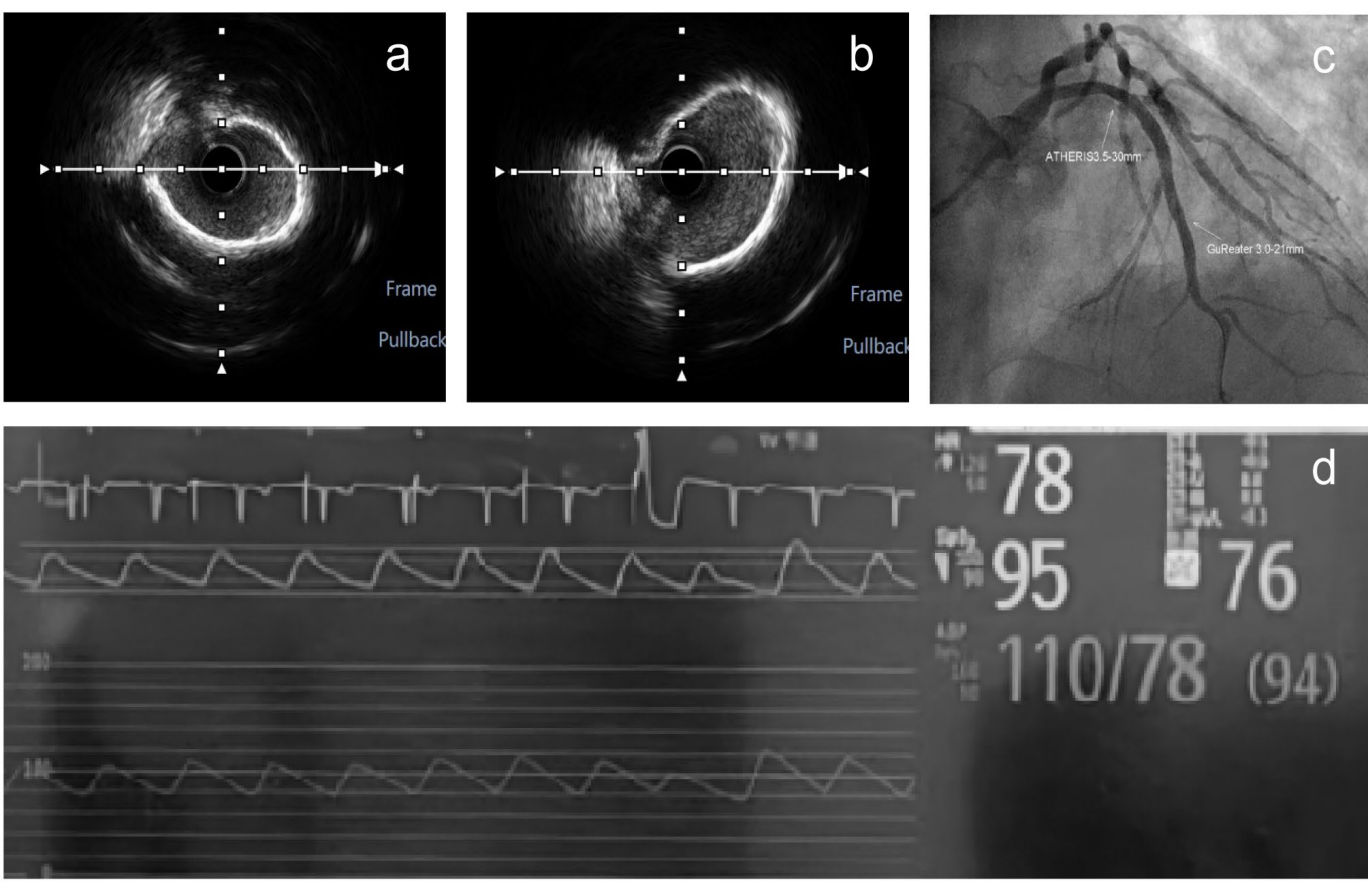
Auxiliary examinations related to intravascular lithotripsy. a is an image of intravascular ultrasound before intravascular lithotripsy. b is an image of intravascular ultrasound after intravascular lithotripsy. c marks the location of the stent (3.0 mm*21 mm) and drug balloon (3.5 mm*30 mm). d shows a ventricular capture
